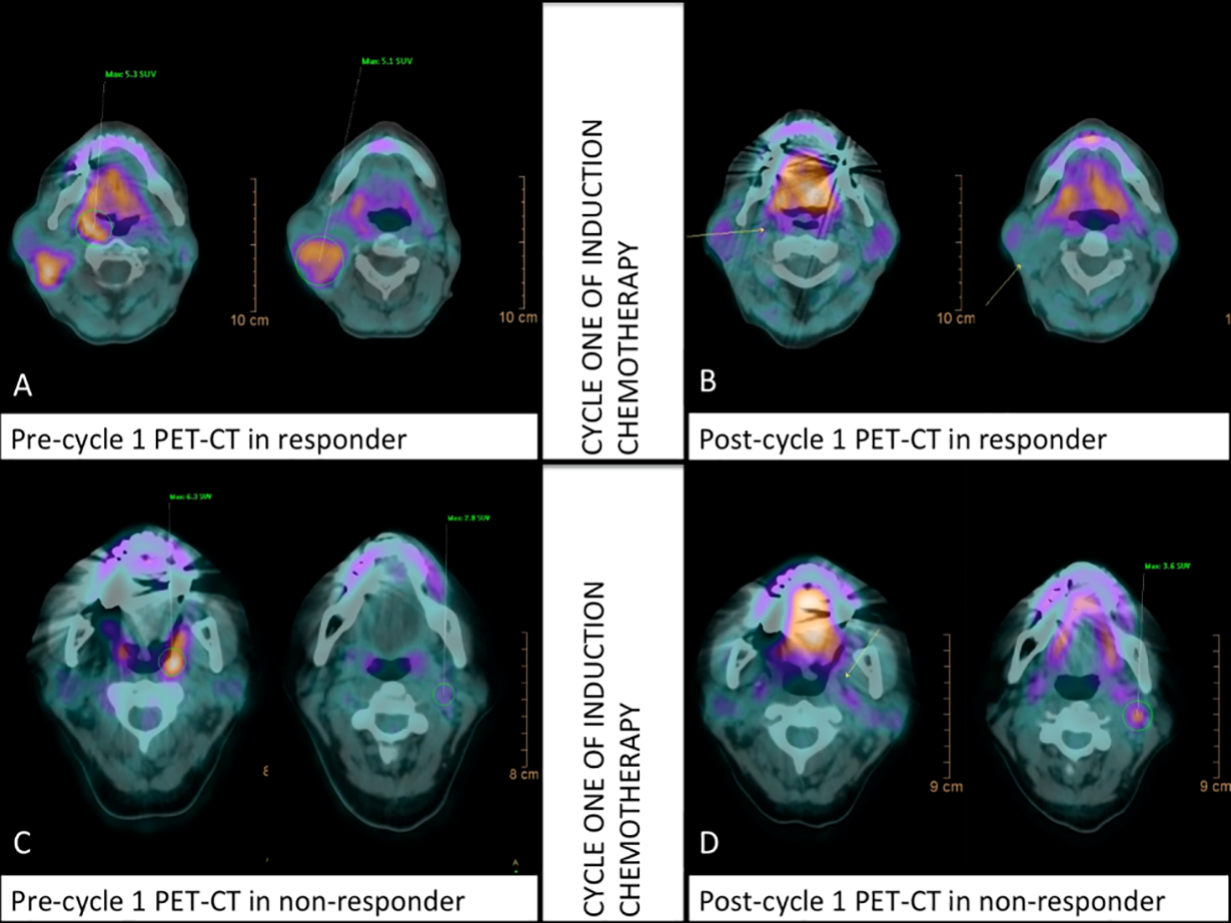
Two patients presenting metabolic responsive and non-responsive tumors/ neck lymph node after once IC cycle. Pretreatment PET/CT(A) and post-treatment PET/CT(B) in patient harboring oropharyngeal tumor with complete metabolic response at 14^th^ day, cycle 1 of induction chemotherapy (SUV variation from 5.1 to 0.0 and 5.3 to 0.0 in lymph node and primary tumor respectively). Pretreatment PET/CT(C) and post-treatment PET/CT(D) in patient harboring non-responsive oropharyngeal tumor with Increased metabolic response at 14^th^ day, cycle 1 of induction chemotherapy (neck lymph node SUV variation from 2.8 to 3.6, despite achieving a complete metabolic response in primary tumor).
